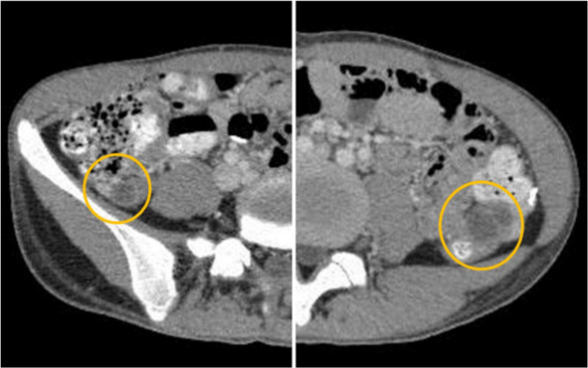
CT showed the ovaries transposed to the level of the anterior superior iliac spine, anterior to the psoas muscle
